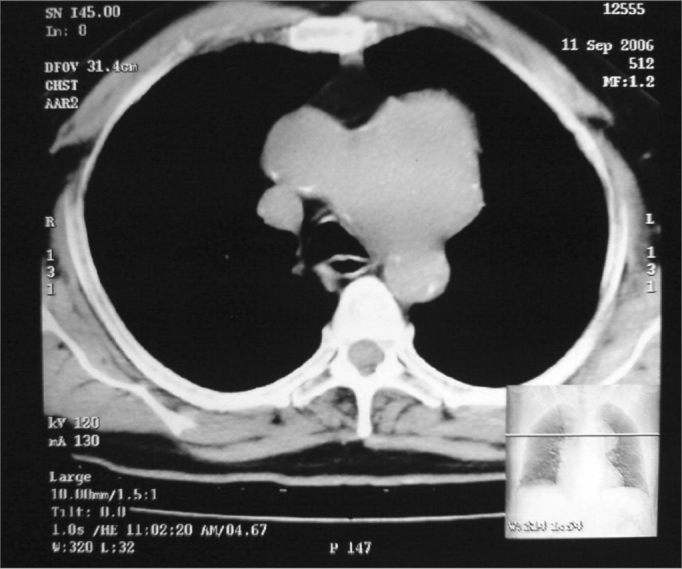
Plain CT scan chest, axial view showing large lobulated mass in the anterior mediastinum.
